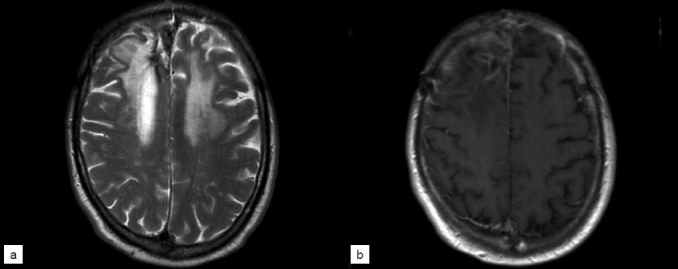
T2 (a) and T1 post-contrast (b) MRI obtained 12 months after craniotomy, showing near-resolution of contrast enhancement with continued evolution of the T2 signal abnormality in the bilateral frontal lobes
